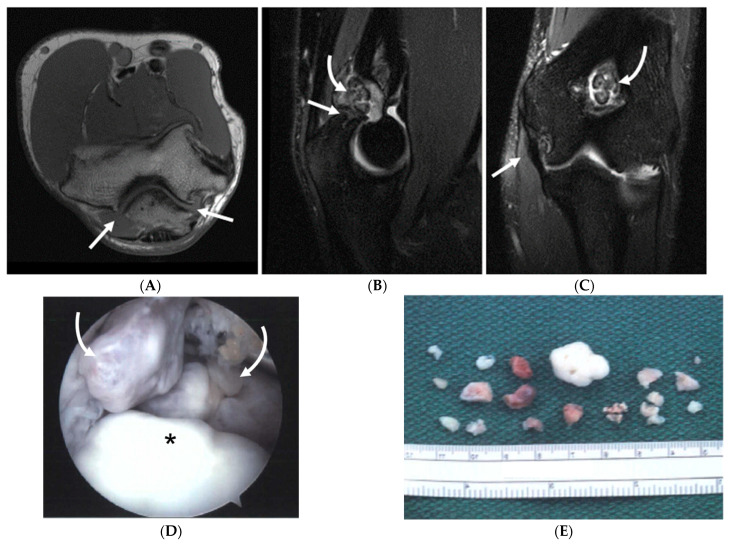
A 19-year-old male collegiate right-hand-dominant baseball pitcher with mechanical symptoms in the right elbow for 2–3 years and an inability to extend elbow. (A) Axial T1-weighted image demonstrates joint space narrowing in the posterior humeroulnar joint, with prominent subchondral sclerosis and small marginal osteophytes (arrows). (B) Sagittal fat-saturated T2-weighted image demonstrates multiple joint bodies in the olecranon fossa (curved arrow) and fragmentation of the olecranon tip (arrow). (C) Coronal fat-saturated T2-weighted image demonstrates marked thickening of the anterior band of the ulnar collateral ligament (arrow). Joint bodies are again seen in the olecranon fossa (curved arrow). (D) Arthroscopic image demonstrating hypertrophy of the olecranon process (asterisk) and multiple joint bodies (curved arrow). (E) Photograph showing the multiple joint bodies retrieved at surgery.
